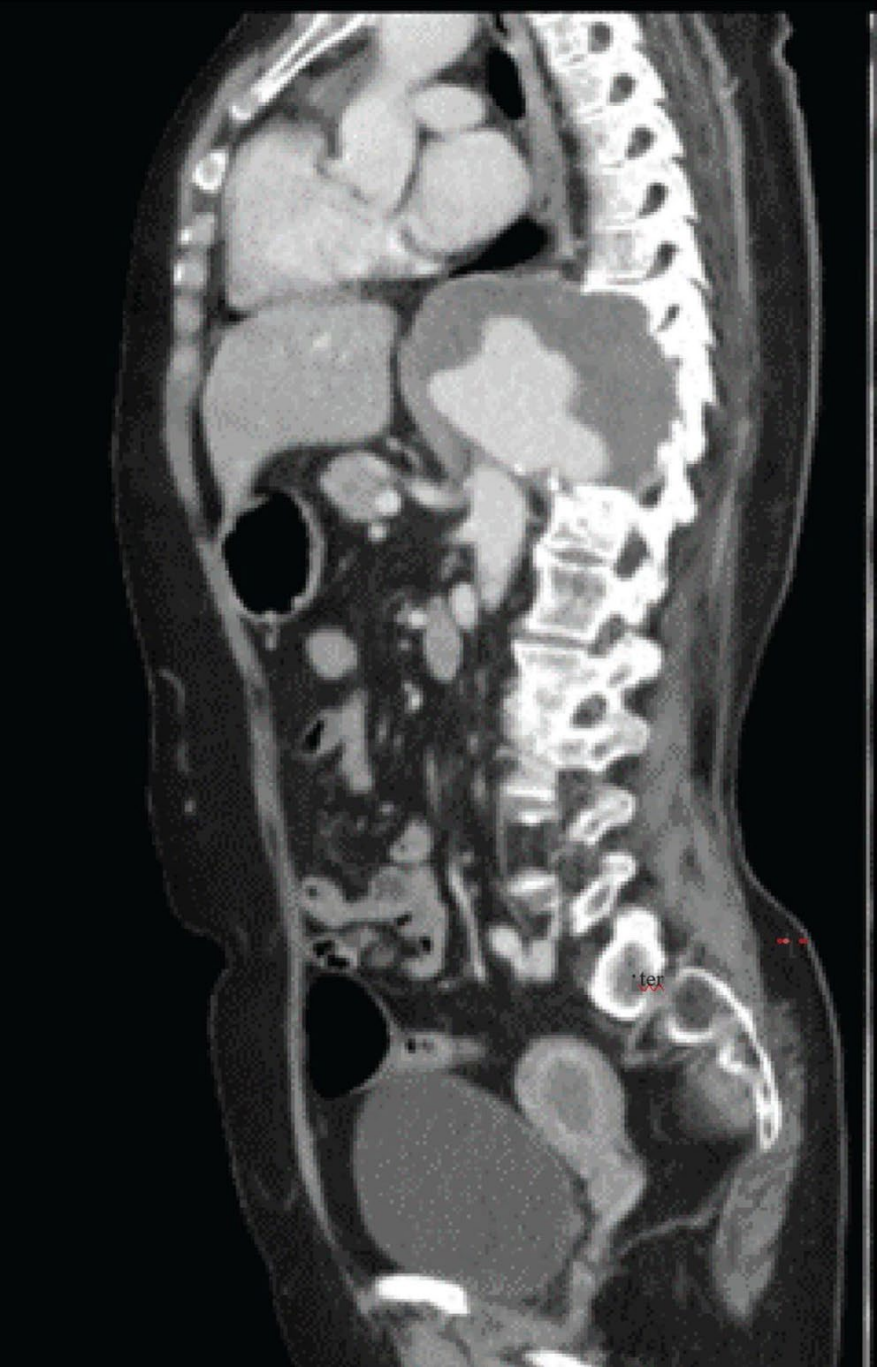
Sagittal CT close‐up view focusing on the thoracic vertebrae. T9 to T11 are markedly collapsed, with partial involvement of T8. The anterior vertebral cortex is eroded, indicating pressure effects from chronic aneurysmal expansion.
